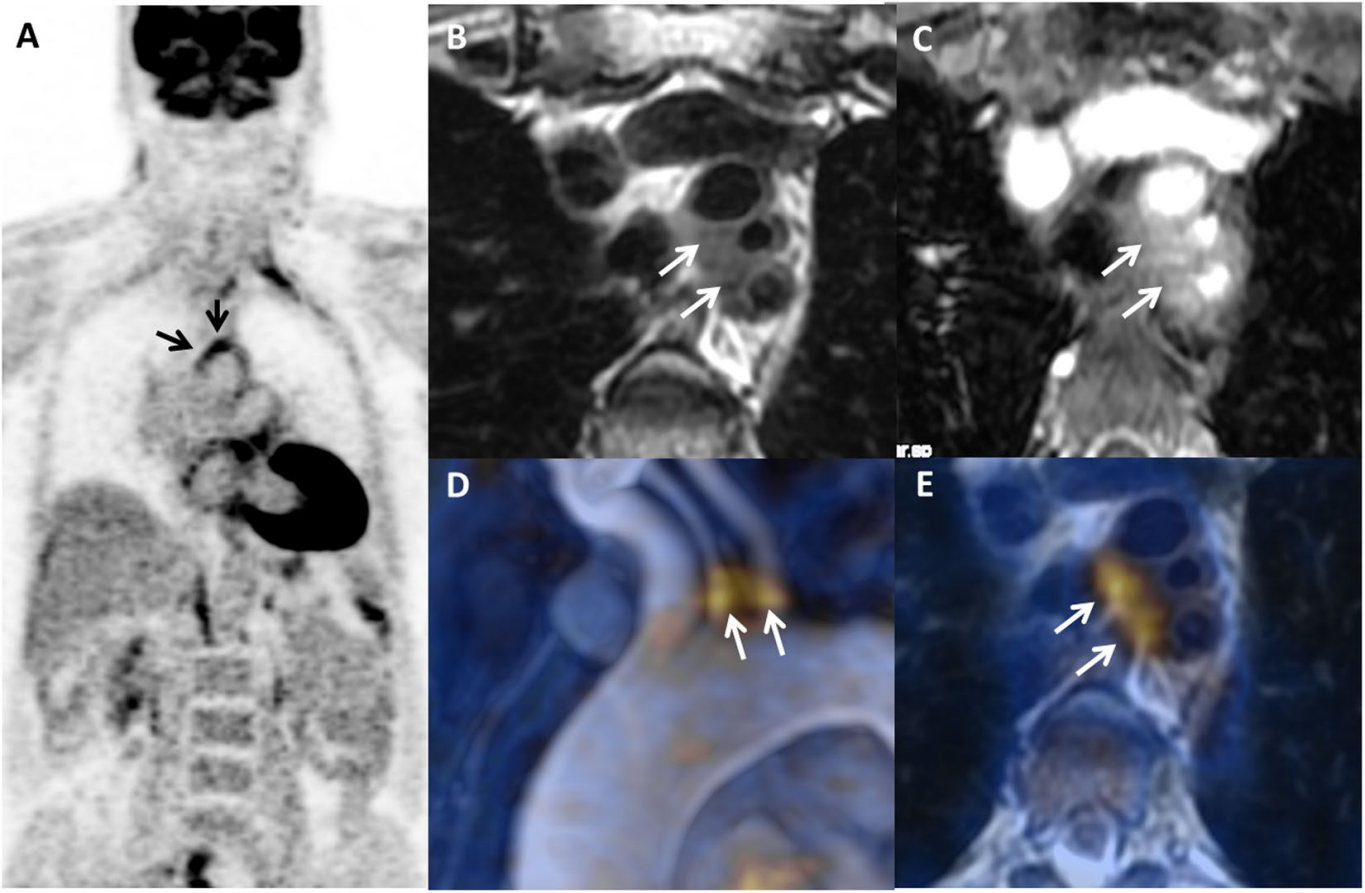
Case of Takayasu arteritis (TA) in a 45-year-old female with arthralgia, vascular claudication, and acute phase reactants refractory to steroids and methotrexate. PET/MRI ((A) coronla PET, (B) T2-weighted image, (C) post-contrast T1-weighted image, (D) fusion MR angiography/PET, (E) fusion PET/T2-weighted image) showed an inflammatory pattern with clear uptake (grade 3) at the origin of supra-aortic vessels associated with arterial wall thickening on T2-weighted image (A, arrows) and wall enhancement (B, arrows). Fusion images (C,D) show excellent co-registration of FDG uptake and MR findings.
